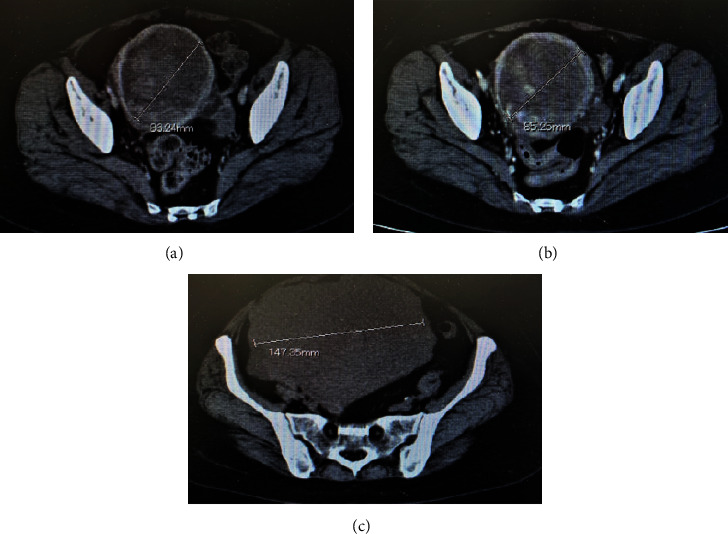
Preoperative pelvic CT findings: (a) 1 year and 6 months after initiation of treatment: myoma fibroids were noted; (b) 1 year and 10 months after initiation of treatment: the patient experienced abdominal pains, and imaging diagnosis demonstrated an infection of a degenerated myoma uteri fibroid; (c) 2 years after initiation of treatment: elevated serum D-dimer and LDH were noted, and imaging diagnosis noted a differential diagnosis of uterine sarcoma or uterine metastasis from lung cancer.
